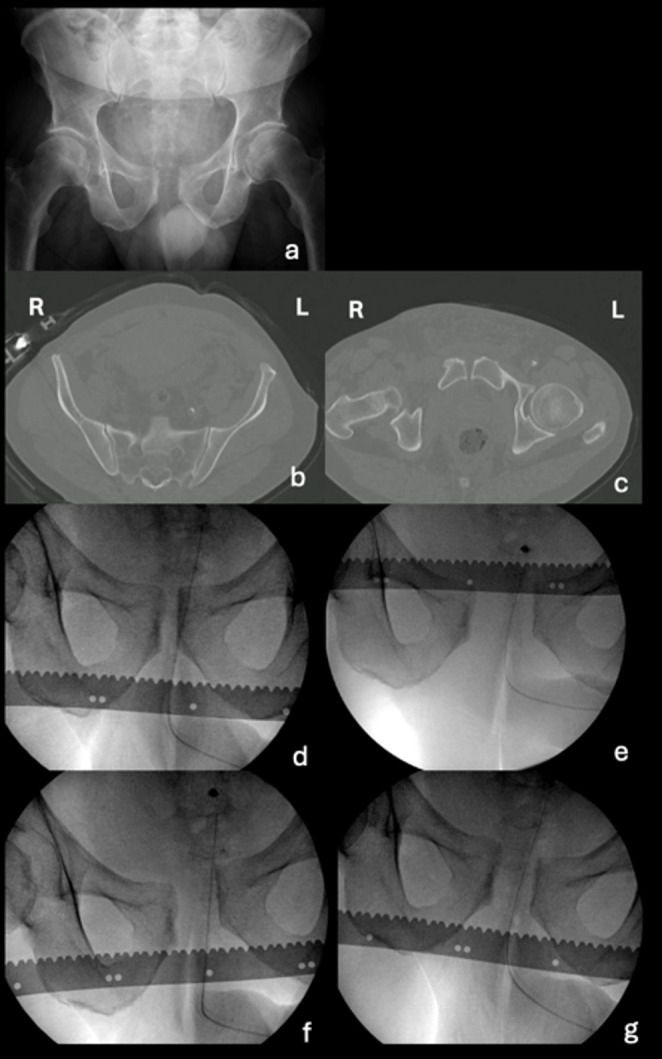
Case 1, a AP static radiograph, (b) CT scan showing the sacroiliac joint, (c) CT scan showing pubic symphysis, (d) AP EUA image in endorotation stress (lateral compression), (e) AP EUA image in exorotation stress (frog position), (f) AP EUA image in push right leg/ pull left leg stress (rotational instability), (g) AP EUA image in push left leg/ pull right leg stress (rotational instability)
